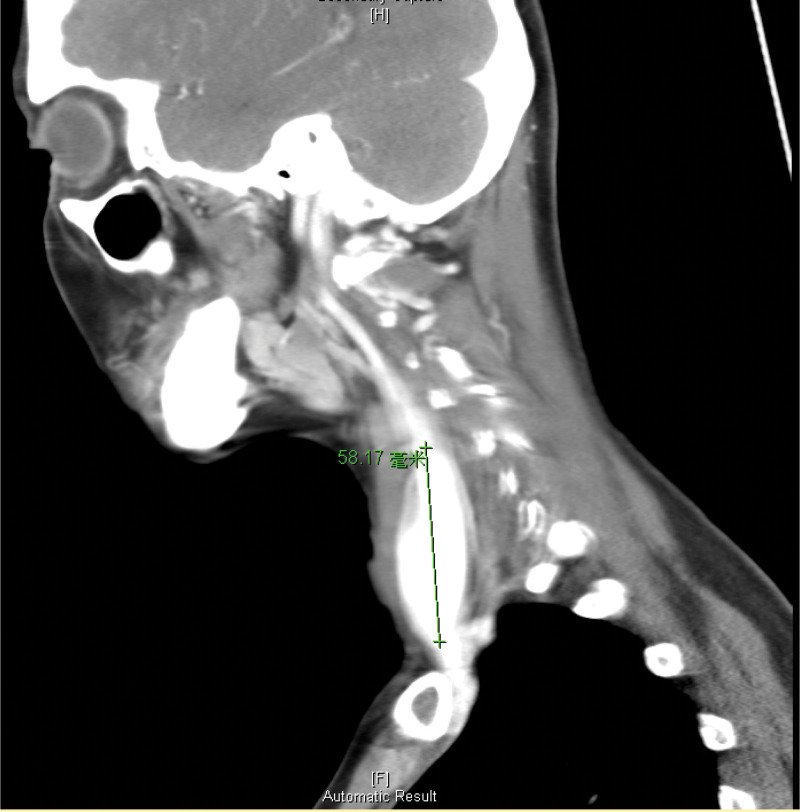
Sagittal view of head and neck CT showed a right fusiform internal jugular vein aneurysm, 58.17 mm in maximum height. CT = computed tomography.
